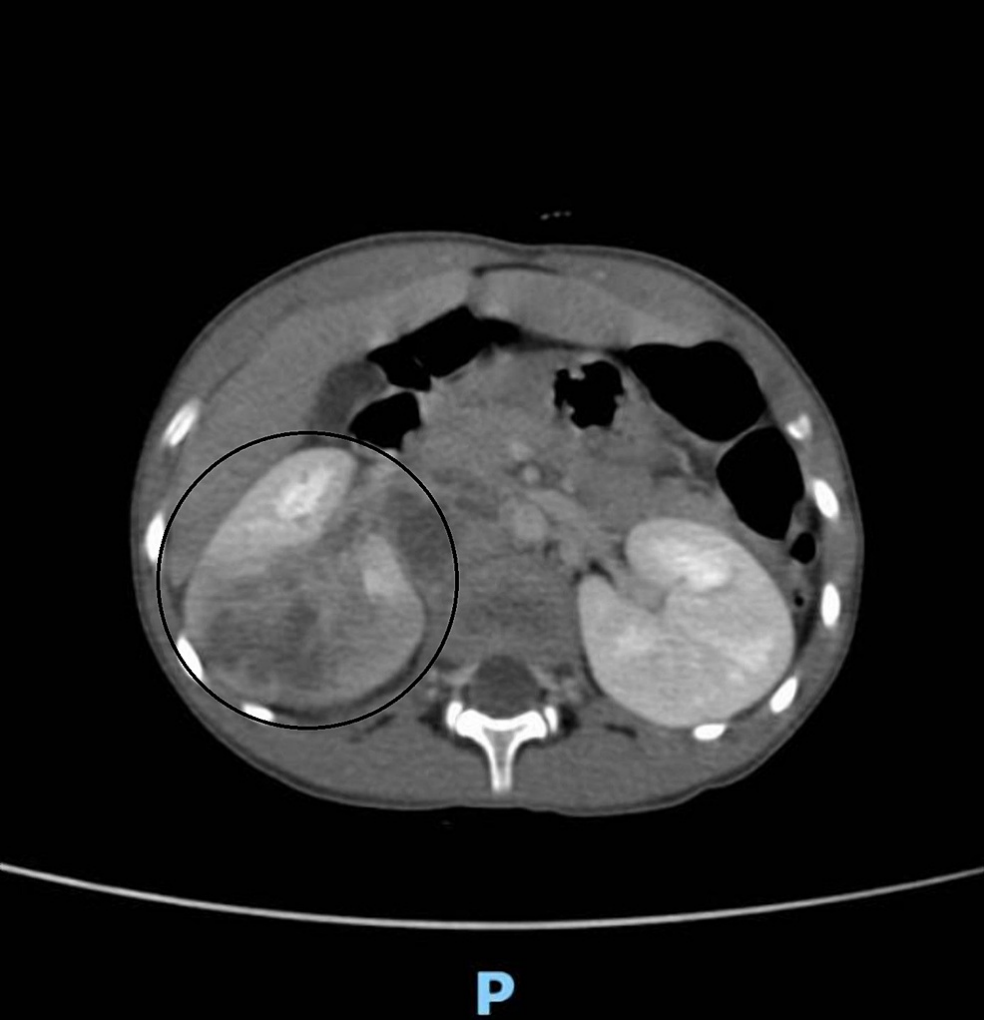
Transverse view of the patient’s abdominal computed tomography revealing an upper right polar renal tumor process with isodense contours, enhancing heterogeneously after injection of contrast agent
